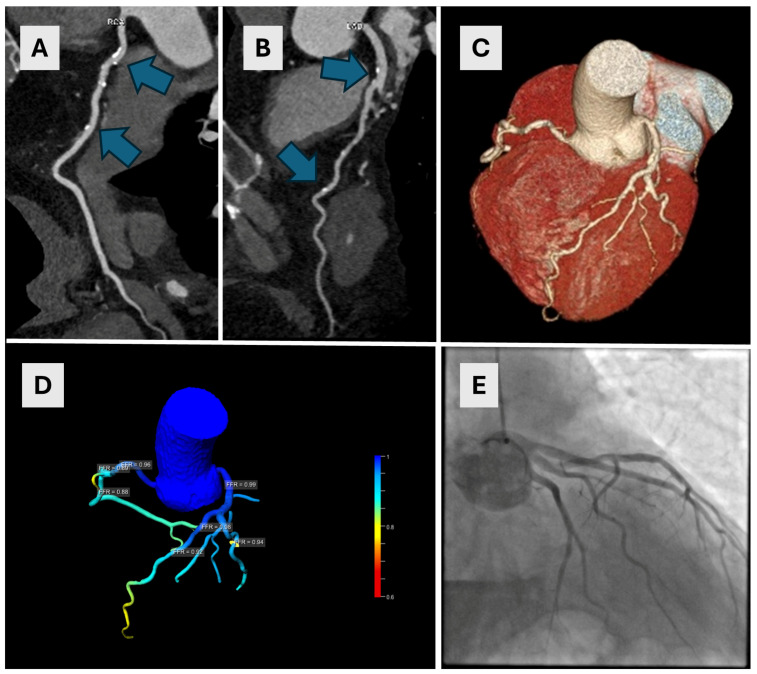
A 54-year-old male underwent CCTA for suspected ischemic heart disease. The CAC score was 417. Calcified plaques were found in both the RCA ((A)—arrows) and LAD ((B)—arrows) causing moderate stenoses (50–52% in the LAD and 63% in the proximal RCA). Image (C) shows a VRT reconstruction image of coronary arteries with multiple small plaques on LAD. A cFFR test showed no significant stenoses (D) which was subsequently confirmed on ICA (E). CCTA—coronary computed tomographic angiography, CAC—coronary artery calcium, RCA—right coronary artery, LAD—left anterior descending artery, VRT—volume rendering technique, cFFR—computed fractional flow reserve, ICA—invasive coronary angiography.
